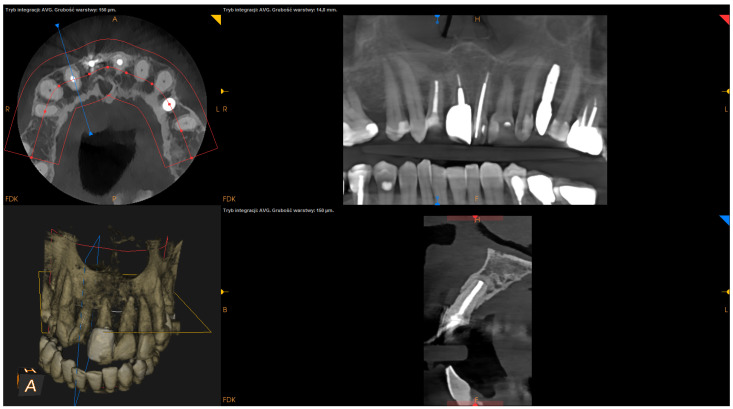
CBCT image (CS 8100 3D Carestream Dental^ⓡ^) example of complete healing based on PENN 3D criteria. Non-English annotations present software technicalities (AVG integration mode and layer thickness).
